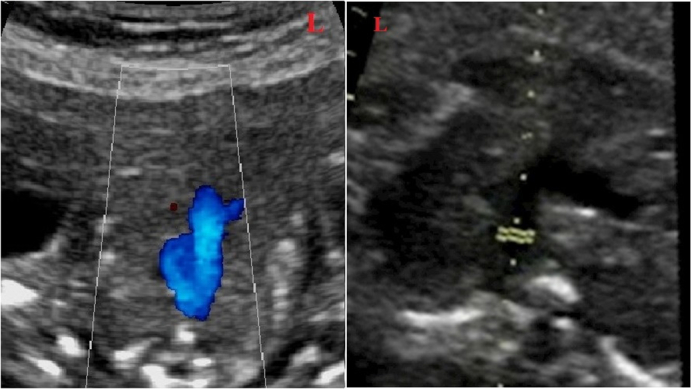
Color and pulsed Doppler examination of DA in the 3-vessel view.
